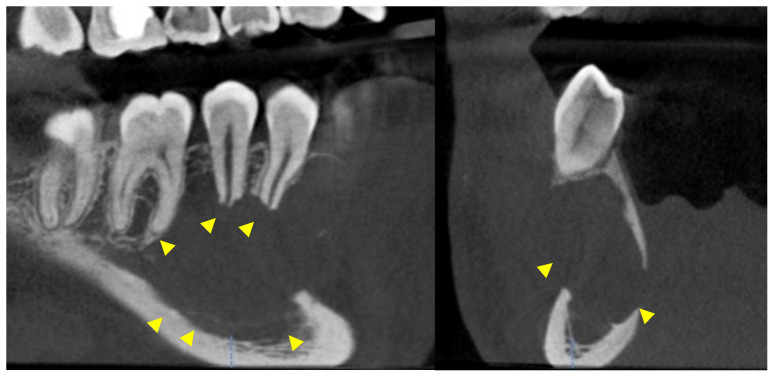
Oblique sagittal and cross-sectional views on a CBCT study. The yellow arrowheads point to the interruption of the lamina dura, the inferior displacement of the inferior alveolar canal, and the thinning/expansion/interruption of the buccal and lingual cortices.
